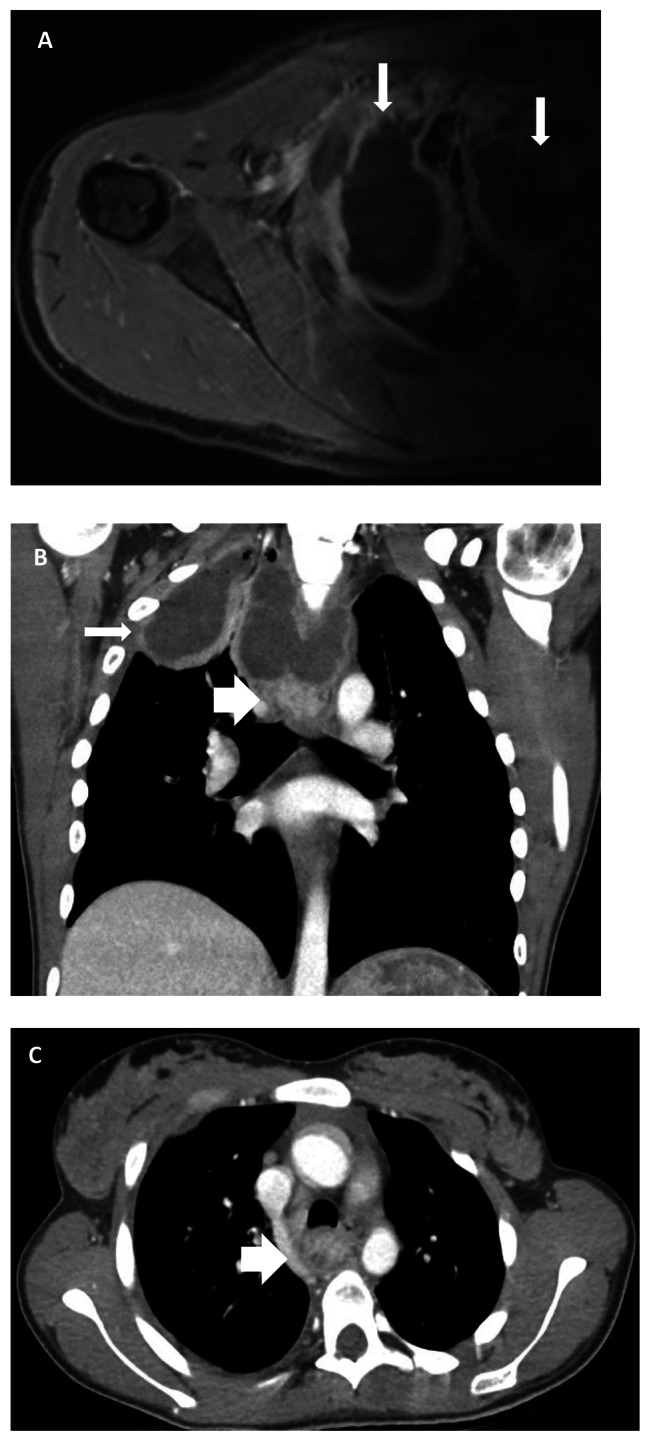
(A) MRI indicating a partially captured, ring enhancing abscess formation in the right hemithorax (narrow arrow). (B?C) CT showing a heterogenously contrasted paratracheal mass in the posterior mediastinum inferiorly adjacent to the abscess formation (broad arrows)
